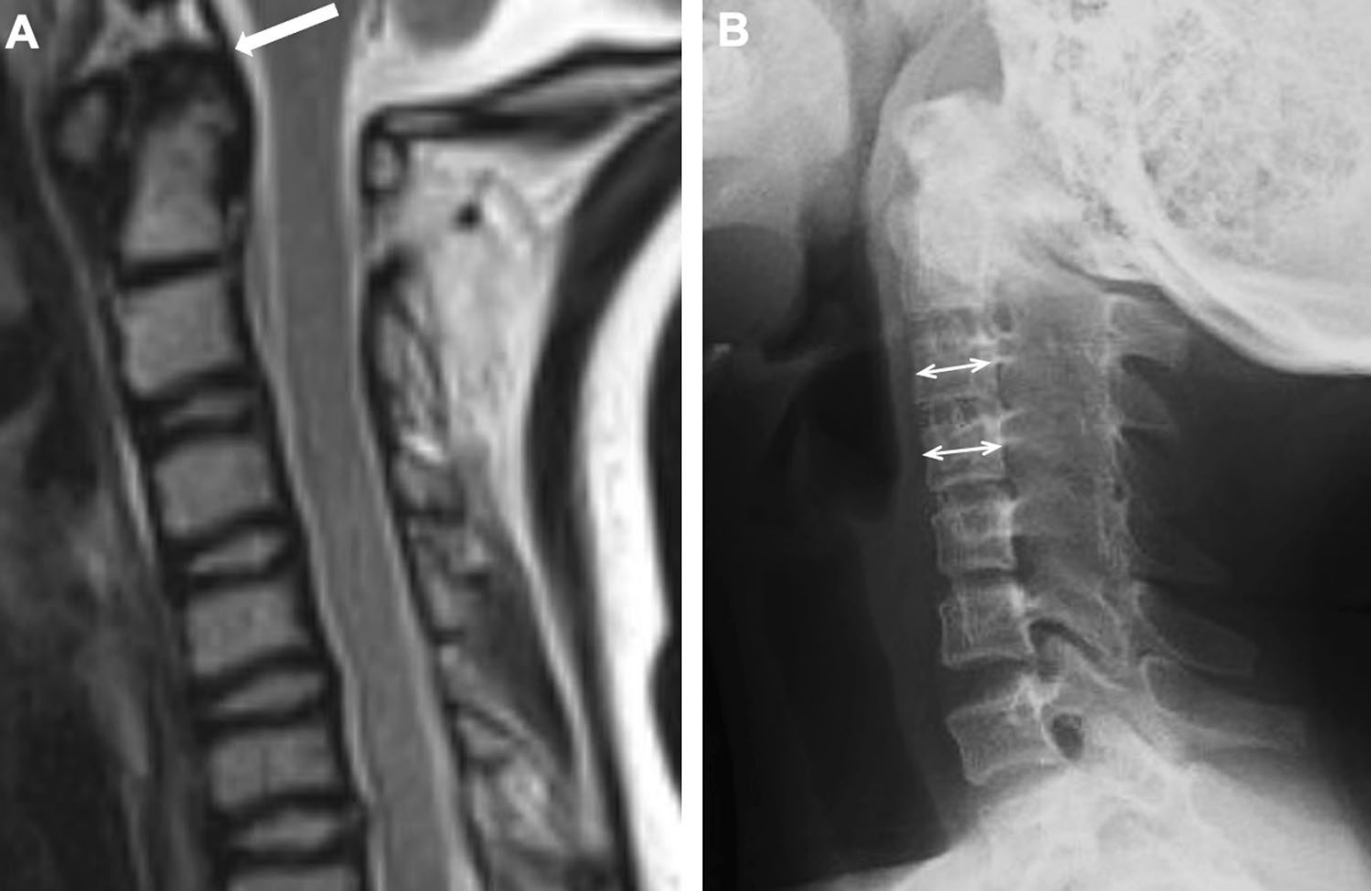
Cervical spine involvement in JIA. A. A 6-year-old girl with oligoarticular type JIA. Synovial hyperplasia of the atlantoaxial joint was depicted as low signal soft tissue prominence of the juxtaodontal region on T2-weighted sagittal image (arrow). B. A 12-year-old girl with systemic JIA. The lateral radiograph of the cervical spine showed ankylosis of the facet joints and reduced anteroposterior diameter of the vertebral bodies (double-headed arrows)
